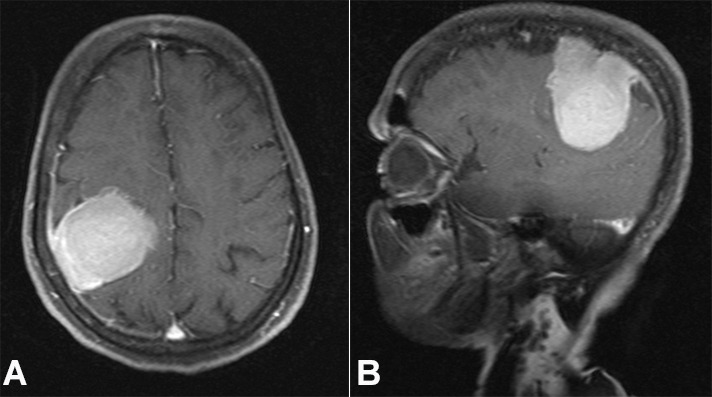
Brain MRI T1 weighted images post-contrast, A – axial plane, and B – sagittal plane, showing fat-saturated and avidly enhancing oval-shaped extra-axial lesion along the left frontoparietal convexity measuring 52mm in the longest dimension. The lesion shows a dural tail characteristic of meningioma.
